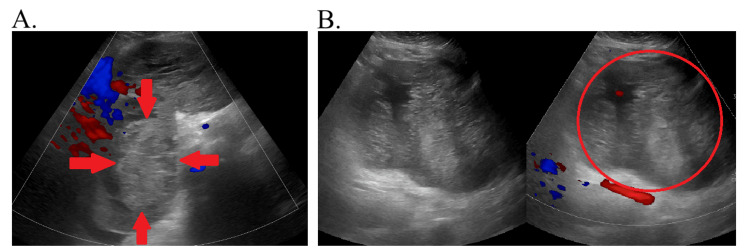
Ultrasound of the abdomen. (a) Ultrasound imaging performed at the prior facility showing an abdominal mass indicated by the arrows. (b) Ultrasound imaging performed at our facility showing an abdominal mass indicated by the circle.
